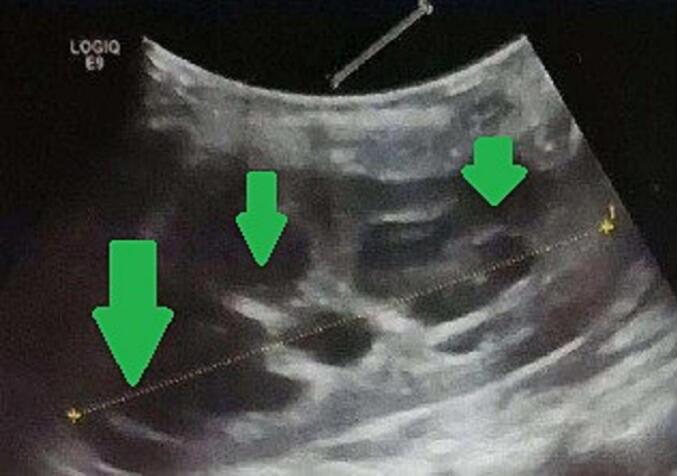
Renal ultrasound showing urinary dilation of the left kidney (Arrow).
